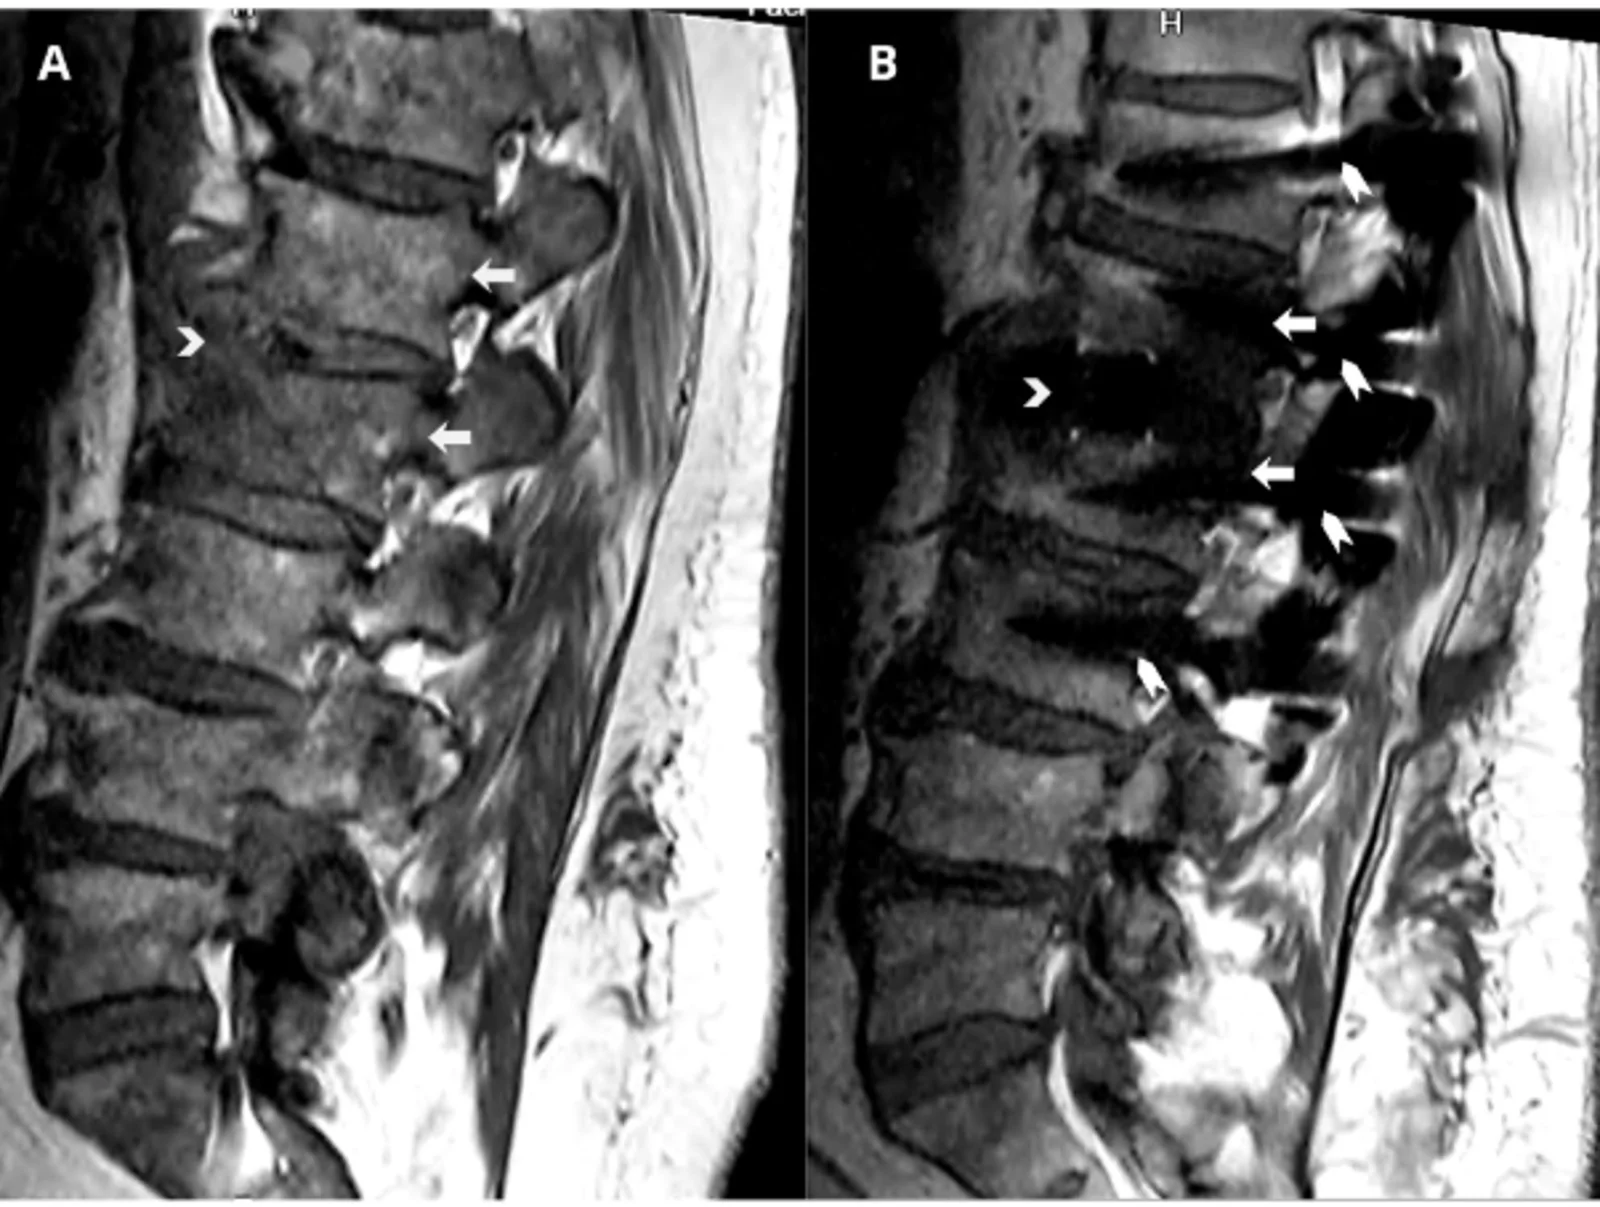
(A) MRI provided by the patient at admission. (B) Follow-up MRI performed before discharge, after the surgical procedure. Short arrowheads indicate changes in the intervertebral space associated with the infection. Long arrows indicate vertebrae L1–L2. Long arrowheads indicate the osteosynthesis material
